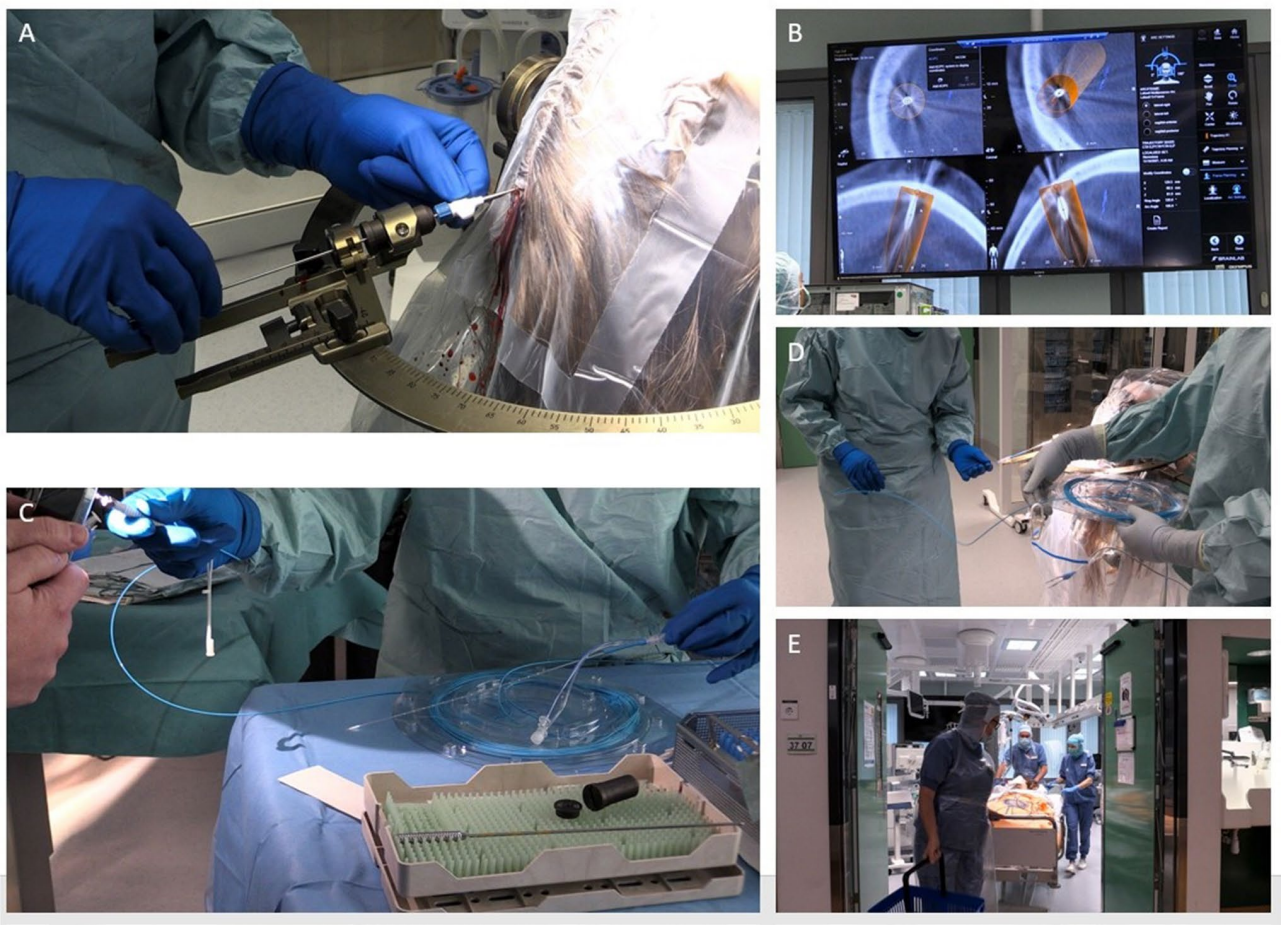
Stereotactic arc and laser catheter insertion A. A small scalp incision is made, followed by drilling of the bone and puncture of the dura. The entry point should have a favorable angulation to the skull, as perpendicular to the bone surface as possible. After the drilling, the rigid stylet for the bone anchor can be passed intracranially B. We verify the trajectory with the O arm, taking images of the rigid stylet before the bone anchor is fixed to the skull. C. The laser fiber is prepared and D the laser catheter is inserted the predetermined distance and locked in place followed by the insertion and fixation of the laser fiber. E The Leksell arc and frame are removed before the patient is transported to the diagnostic MRI suite
